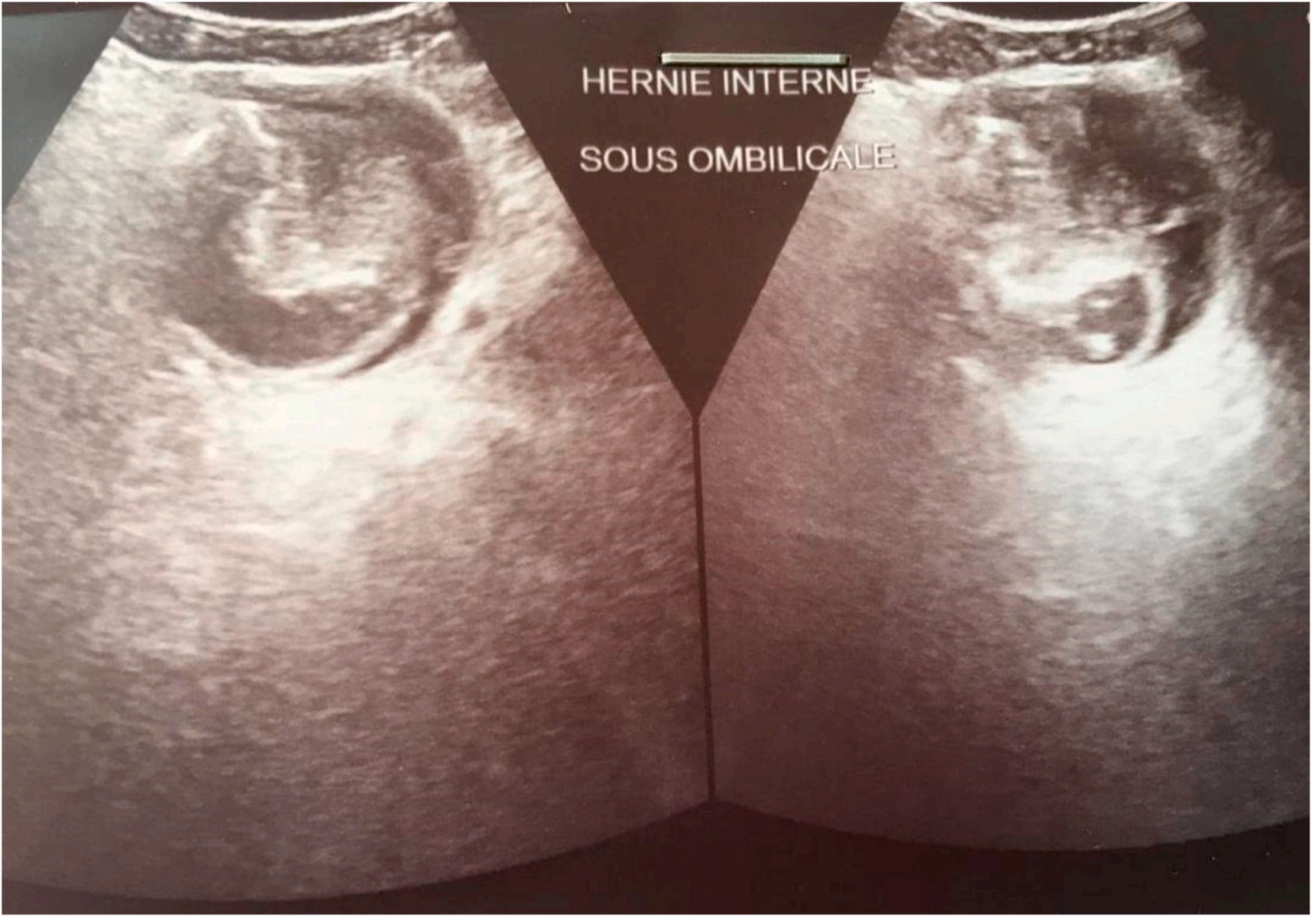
Abdominal ultrasound showing a strangulated internal hernia.
